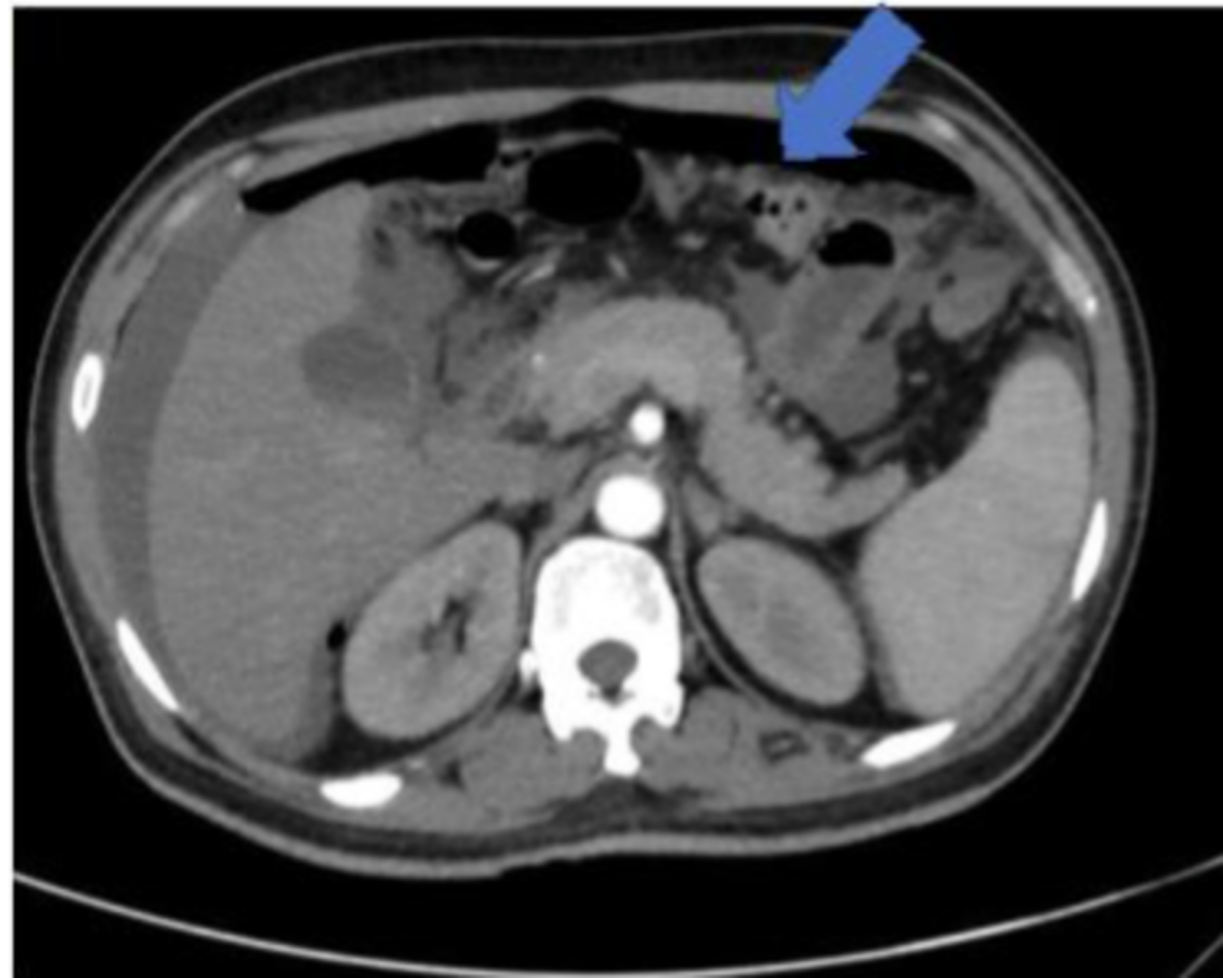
Abdominal CT scan revealing pneumoperitoneum and intraperitoneal fluid secondary to bowel perforation.
